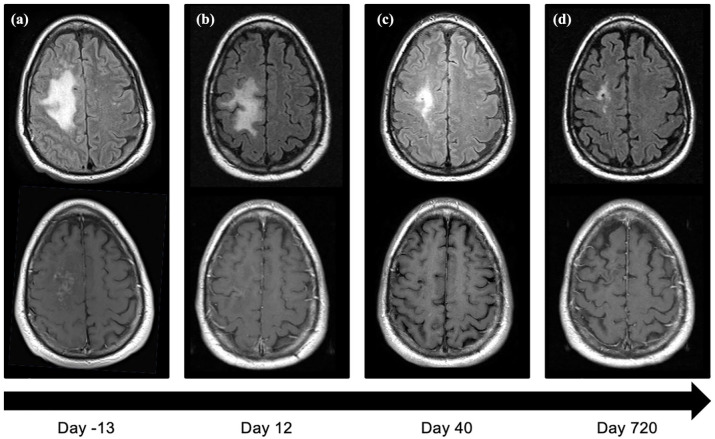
(a) Imaging showing the initial axial fluid-attenuated inversion recovery magnetic resonance imaging (MRI) of the patient’s brain, showing a hyper-intensive lesion in the posterior right frontal lobe. Typical for PML is sparing of the more superficial cortex. (b–d) Images obtained at days 12, 40, and 720 post-treatment initiation, showed significant resolution based on a decrease in the hyperintensity of the lesion. PML, progressive multifocal leukoencephalopathy.
